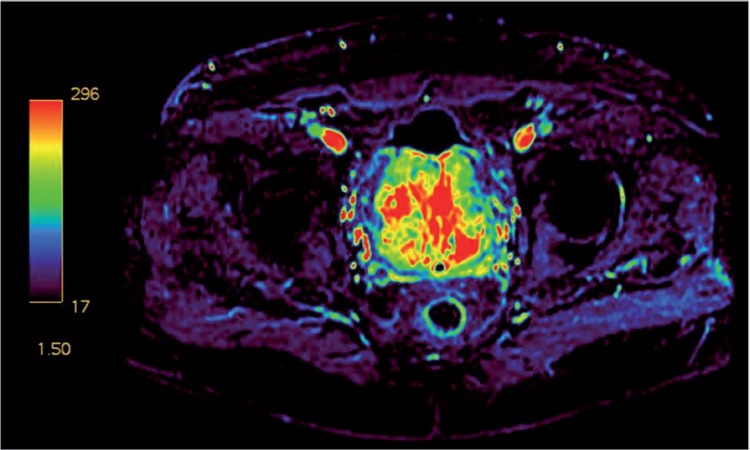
Prostate MRI contrast-enhanced perfusion map showing a markedly increased perfusion.
